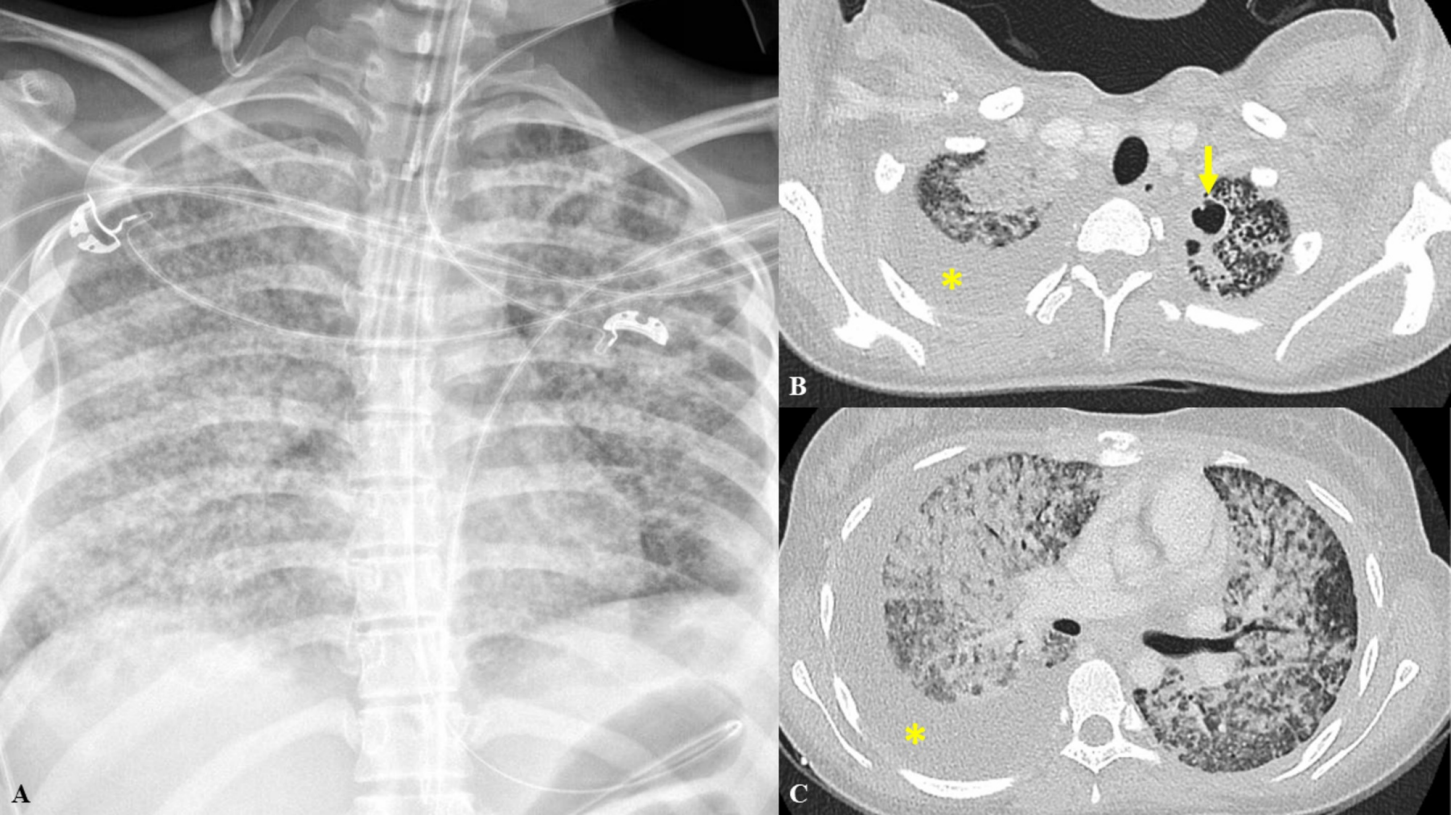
Chest imaging A) Post-intubation chest X-ray showing “severe bilateral lung opacities in a coalescing miliary type appearance. Small bilateral effusions.” B) Select CT Chest image showing “...a thick-walled pleural-based cavitary lesion medial left lung apex measuring 17 x 14 mm…”. Cavitary lesion identified by the arrow. C) Select CT chest image showing “...coalescence of innumerable parenchymal micronodules… Focal pleural-based mass-like opacity lateral left lower lobe measuring 26 x 14 mm…”. Pleural opacity identified by the asterisk CT: computed tomography
